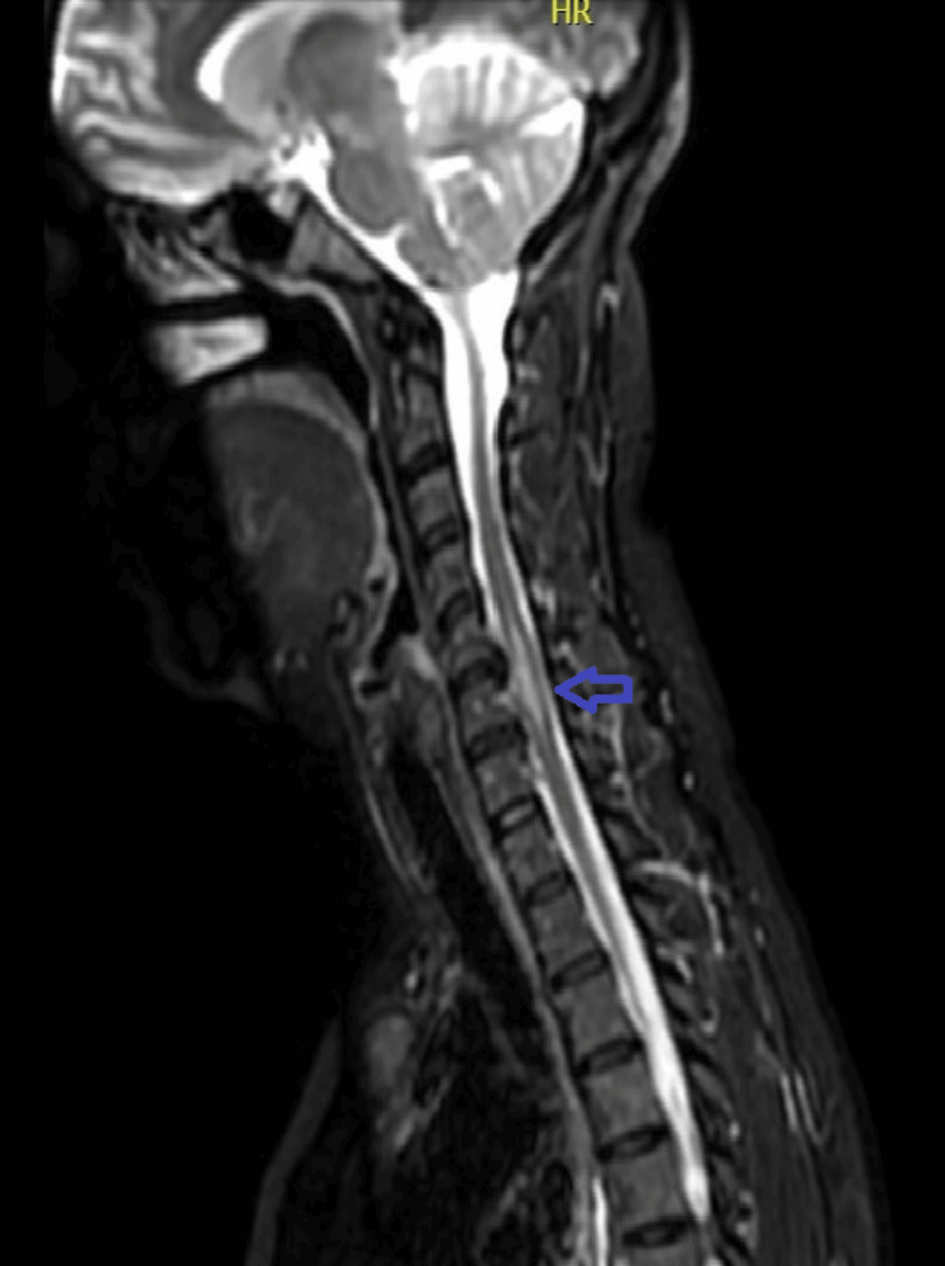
Cervical spine MRI showing TM Sagittal T2-weighted MRI of the cervical spine showing a hyperintense intramedullary lesion extending from C5 to C7 (arrow), with mild cord expansion and no contrast enhancement, consistent with TM. TM: Transverse myelitis
